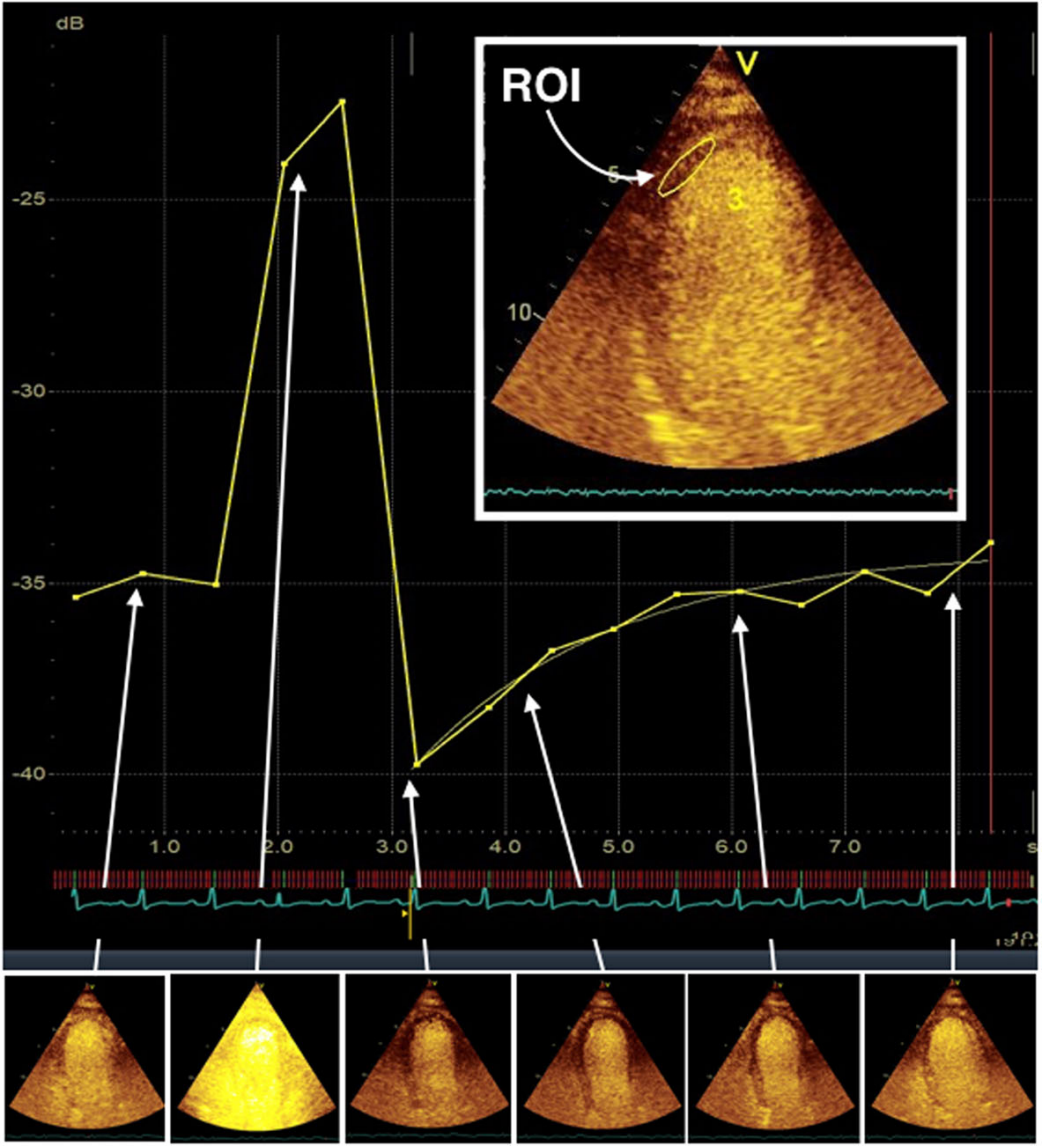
Myocardial contrast perfusion echocardiography (MCPE): quantitative analysis. Echo contrast microbubbles are small enough to pass through the microcirculation, and this feature can be used to estimate myocardial blood flow. A region of interest (ROI) is defined in a myocardial segment and the signal intensity at the plateau and the rate of change at each end-diastolic frame are analysed to estimate myocardial blood flow
